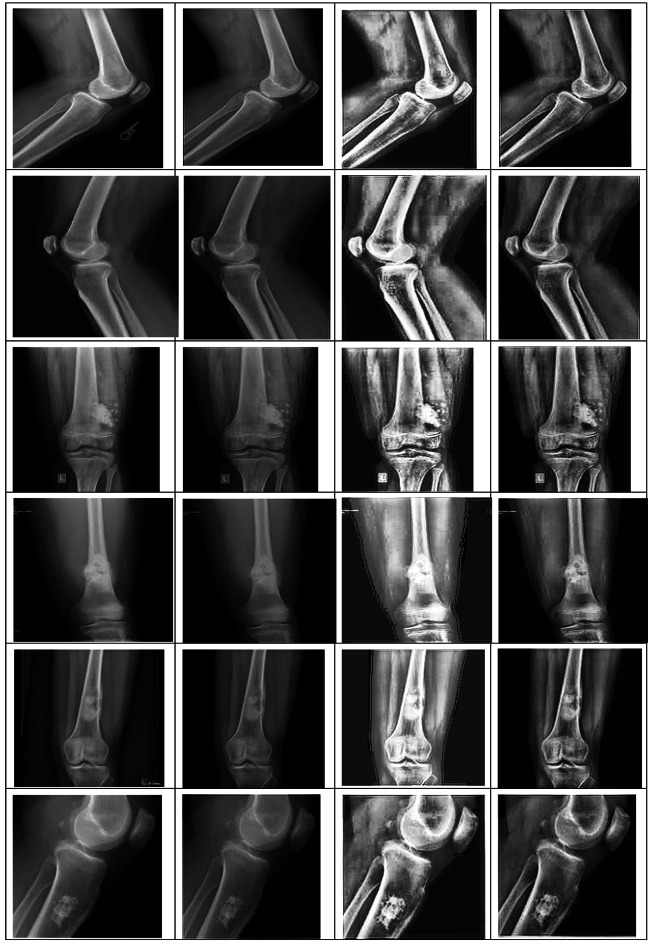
Outcomes of the Weiner filter, histogram equalization, and CLAHE Filter algorithms to enhance the X-ray bone images.
